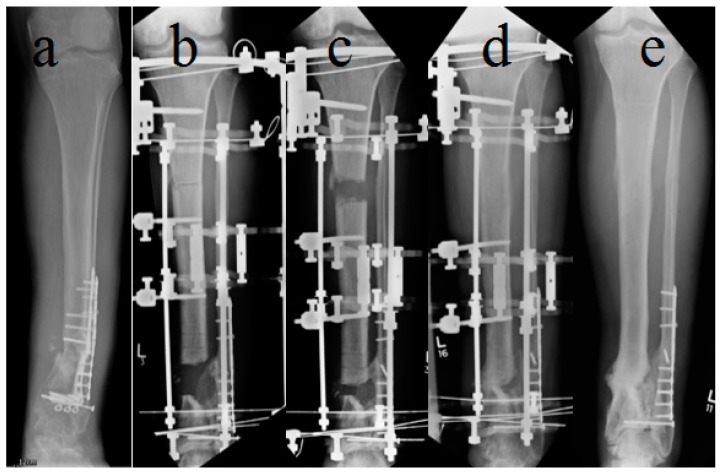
Bone Transport.* (a) Previous open fracture of distal tibia, treated with internal fixation. The wound broke down and he was referred with a mobile infected non-union. Preoperative AP x-ray shows that the lateral tibial plate has broken at the non-union. (b) Four centimetre resection of the non-union after removal of the infected tibial plate. The fibula was intact and well aligned, so a bone transport was preferred. (c) Twenty-six days after surgery. The proximal corticotomy is distracted at 1 mm per day. (d) Six weeks after docking. No surgery was required to secure union at the docking site. (e) Four months after frame removal with well consolidated docking site, regenerate and good alignment.
